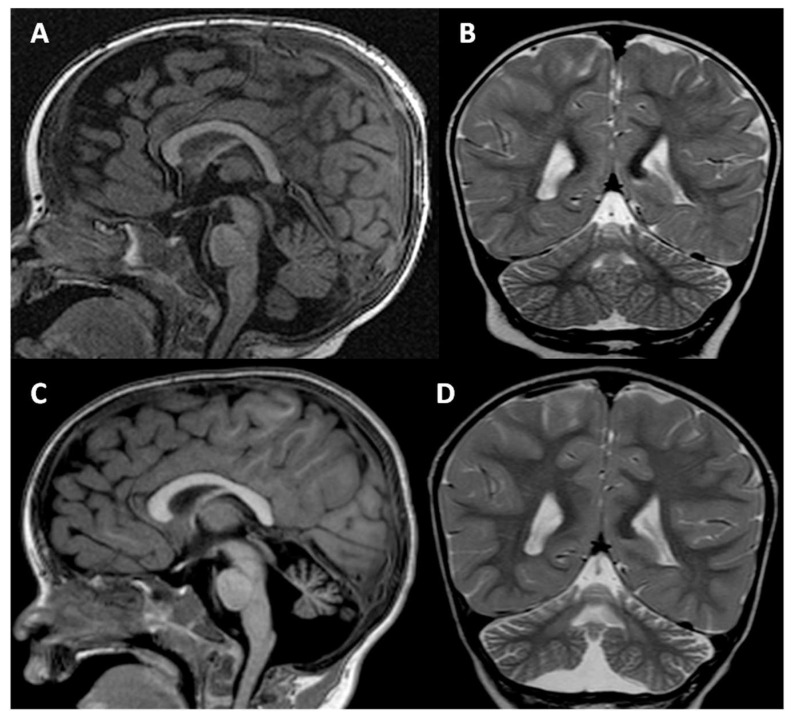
MRI of the brain was performed when the patient was 3 months (A,B) and 3 years old (C,D). Both midline sagittal T1-weighted and coronal T2-weighted images demonstrate a progressive reduction in cerebellar volume, especially of the vermis, with diffuse atrophy of the folia and secondary enlargement of the subtentorial CSF spaces. The *corpus callosum* is normal except for minimal hypoplasia of the rostrum (A,C). Note also the pathological progressive increased hyperintensity on T2-weighted images of the dentate nuclei (B,D).
